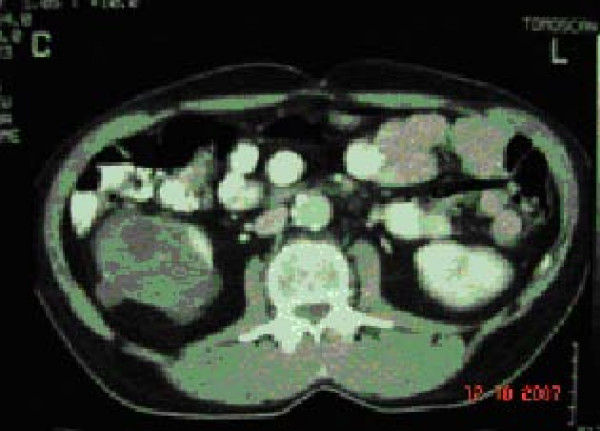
Cystic nephroma: Computed tomography image.
